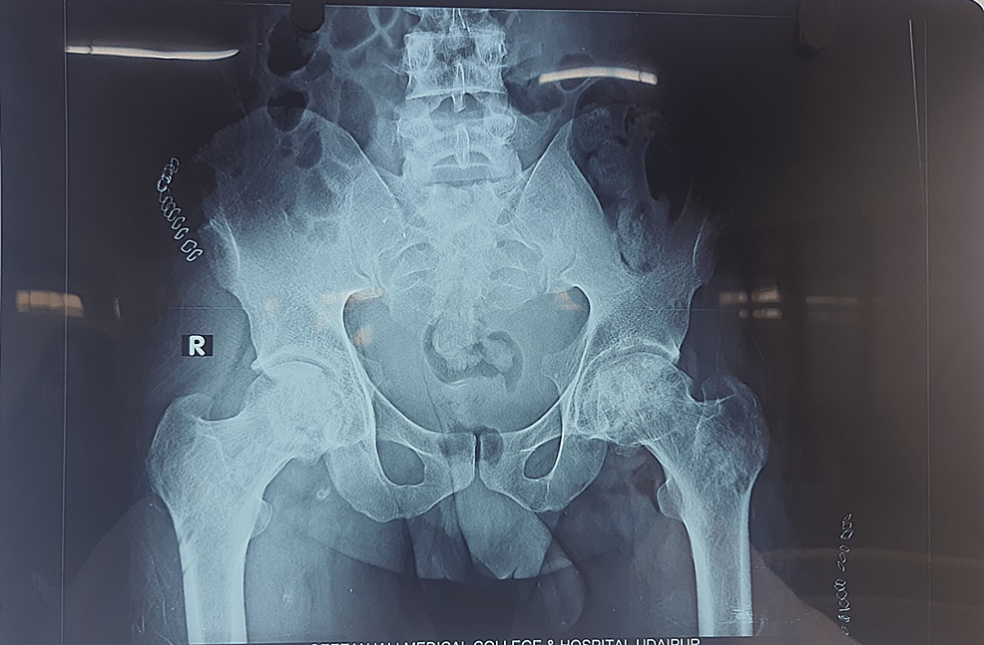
X-ray at three months of follow-up.
